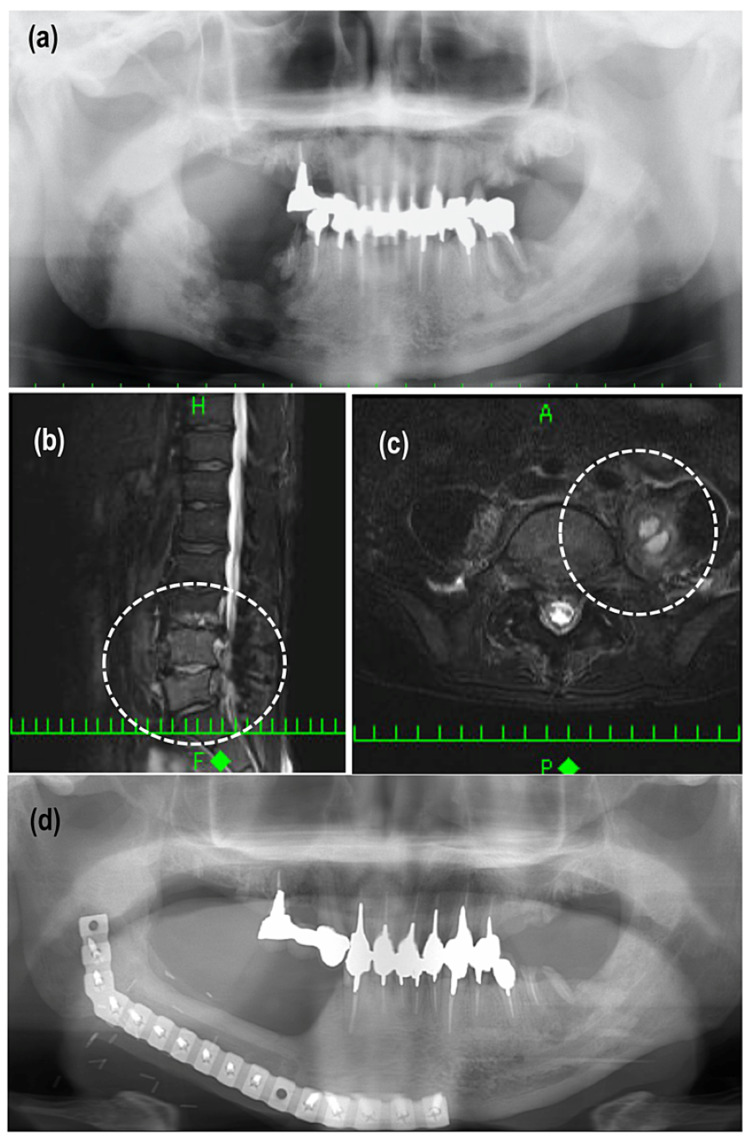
Distant infection in patient 2. (a) Preoperative panoramic radiographic image shows bone destruction close to the inferior border of the mandible. Distant infection occurred 82 days postoperatively. Magnetic resonance imaging revealed (b) pyogenic spondylitis and (c) an iliopsoas abscess. (d) Panoramic radiographic image 5 years after surgery shows excellent bone union.
